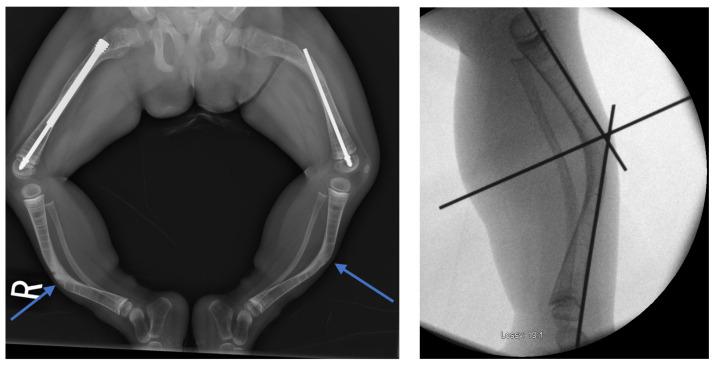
Preoperative and intraoperative X-rays of the lower extremities showing tibia deformities/fractures. Blue arrows indicate the CORAs.
